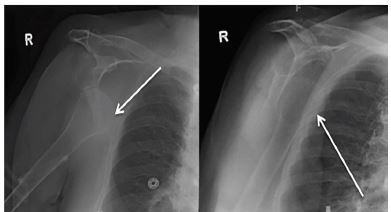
Radiographs demonstrating acute anterior-inferior right shoulder dislocation. White arrows pointing to the humeral head, which is anterior and inferior to the glenoid.
